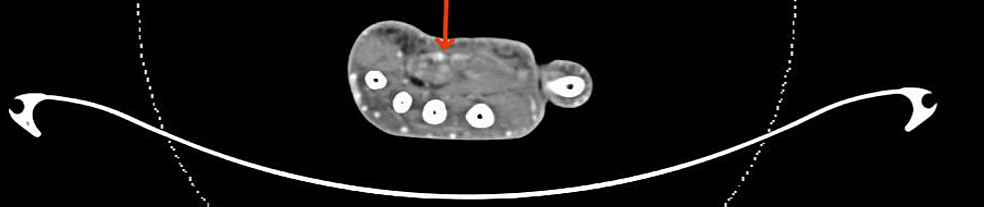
CT angiography The patency of the superficial palmar arch is redemonstrated in a more distal image. The superficial palmar arch is indicated by the red arrow.
